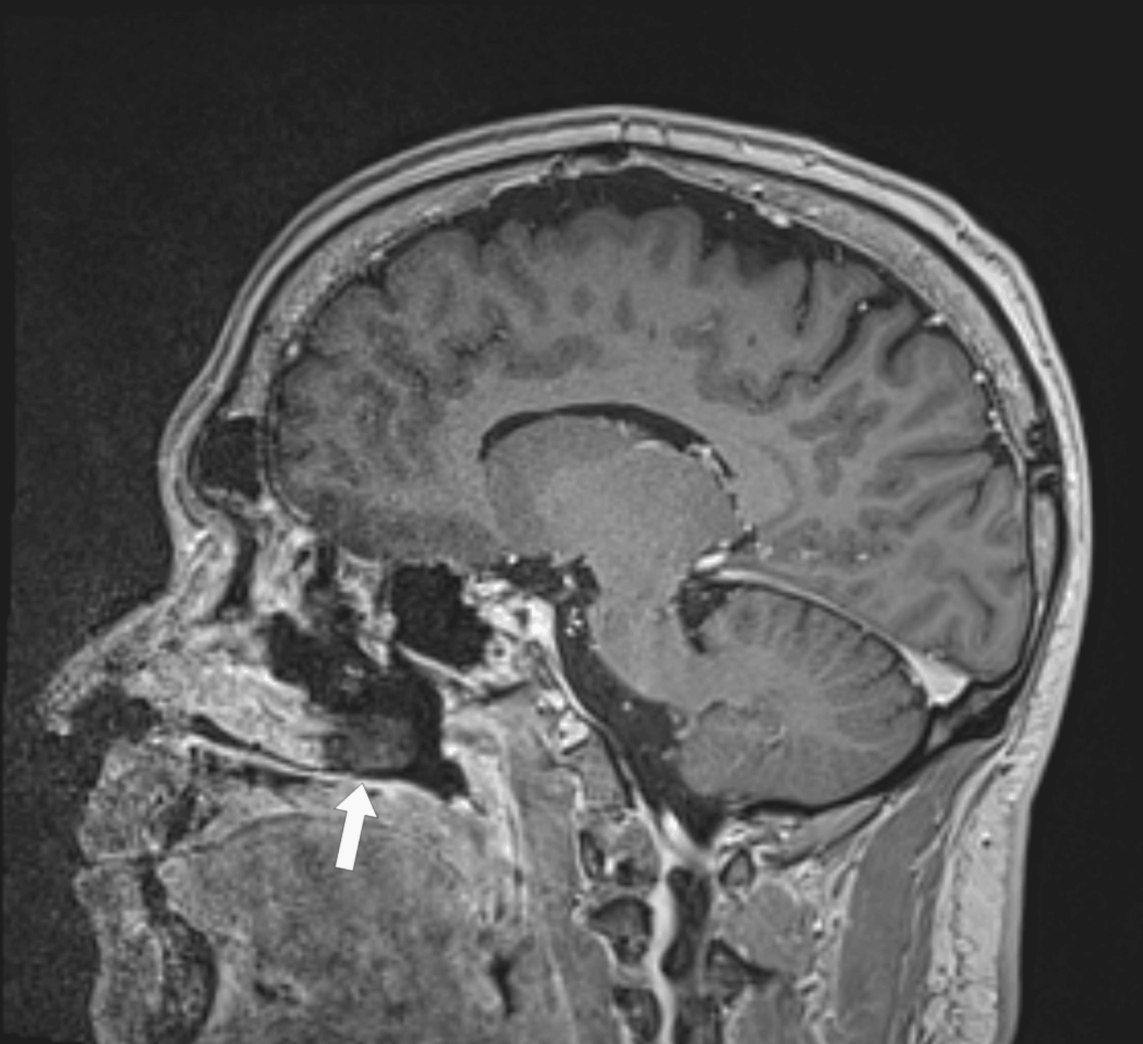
MRI showing hypointensity of the posterior aspect of the left inferior turbinate suggestive of fungal infection and invasion MRI: magnetic resonance imaging
